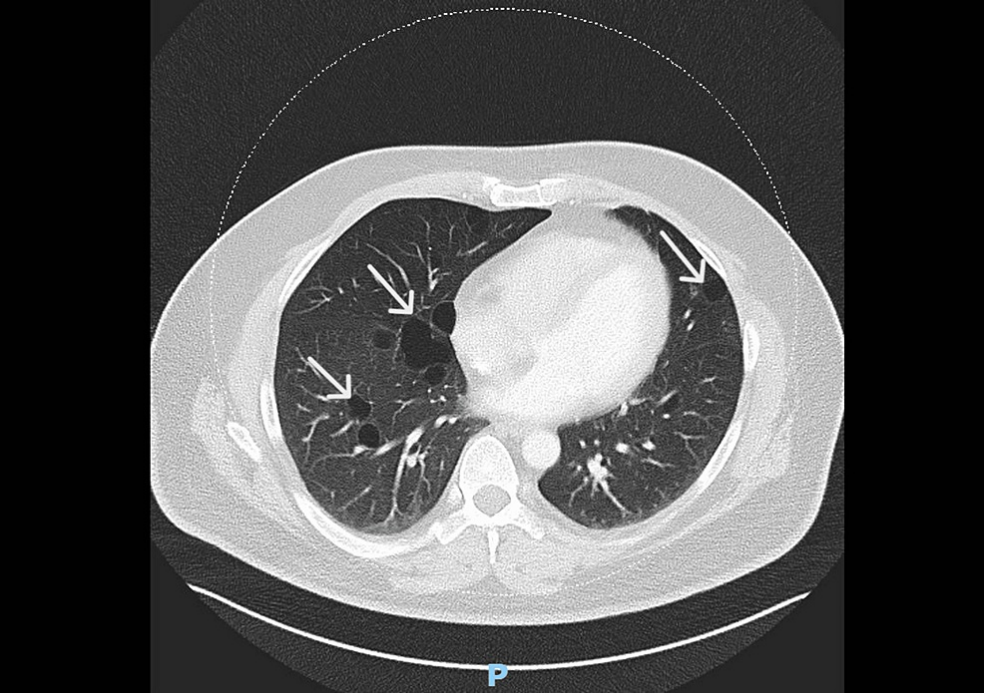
CT scan of the thorax showing multiple thin-walled cysts (arrows) in both lungs of varying sizes CT, computed tomography
